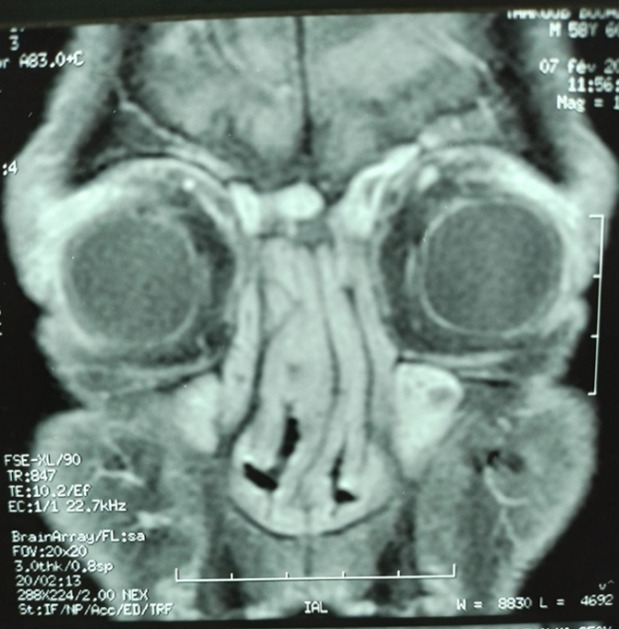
Coronal MRI (T1 sequence) revealing dacryoadenitis
